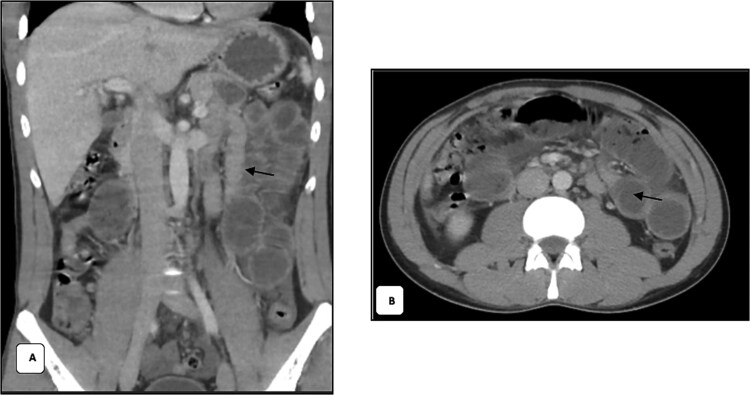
(A) Coronal contrast-enhanced CT shows dilated proximal jejunal loops with air-fluid levels and a clear transition zone in the left lower quadrant (arrow). (B) Axial contrast-enhanced CT shows dilated small-bowel loops with preserved wall enhancement, patent mesenteric vessels and a transition zone in the left lower quadrant (arrow).
